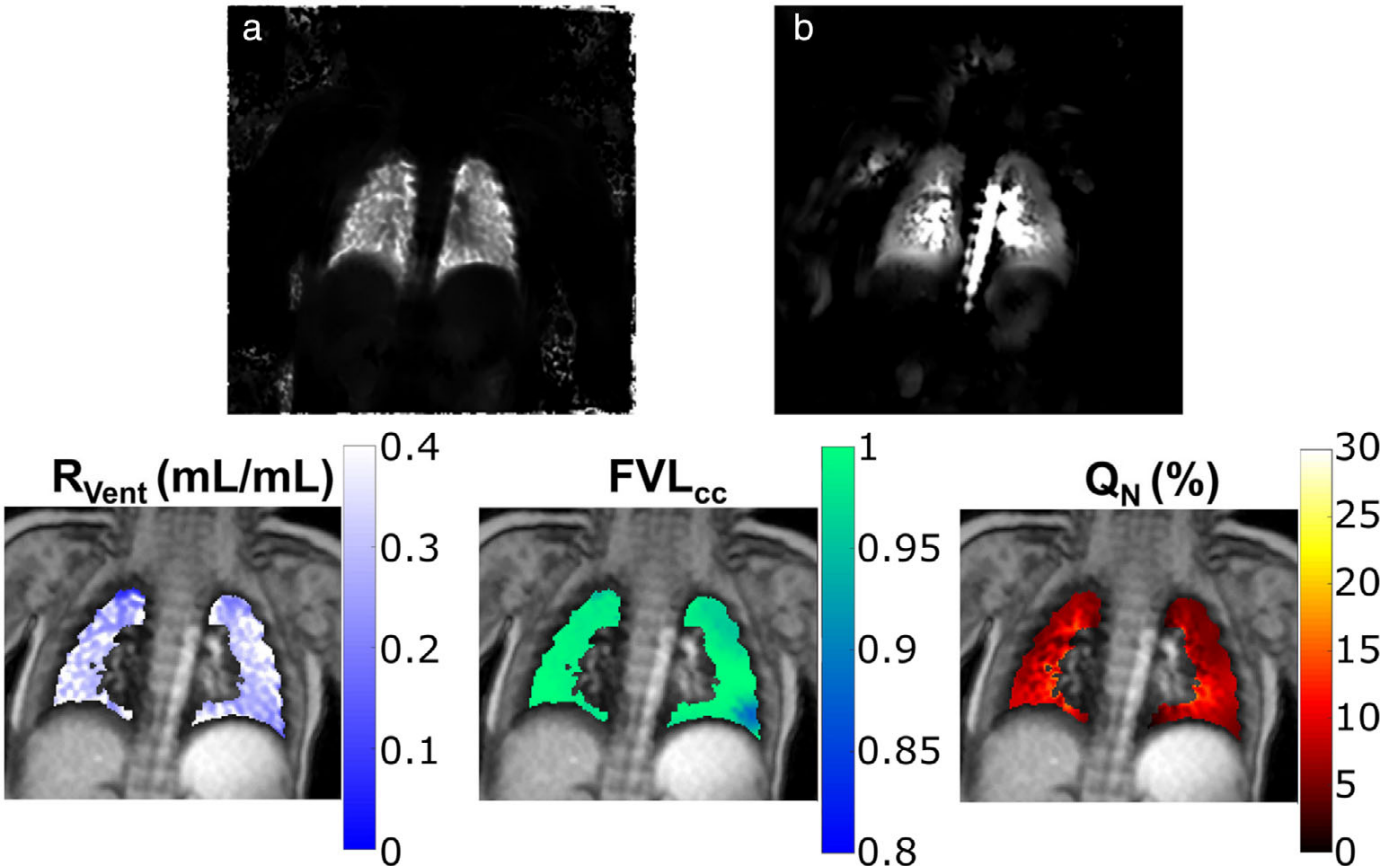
PREFUL MRI results obtained from an 8‐month‐old infant using a 2D gradient echo sequence. Reprinted with permission from Reference 82. (a) Ventilation‐, (b) perfusion‐weighted maps. *R*
